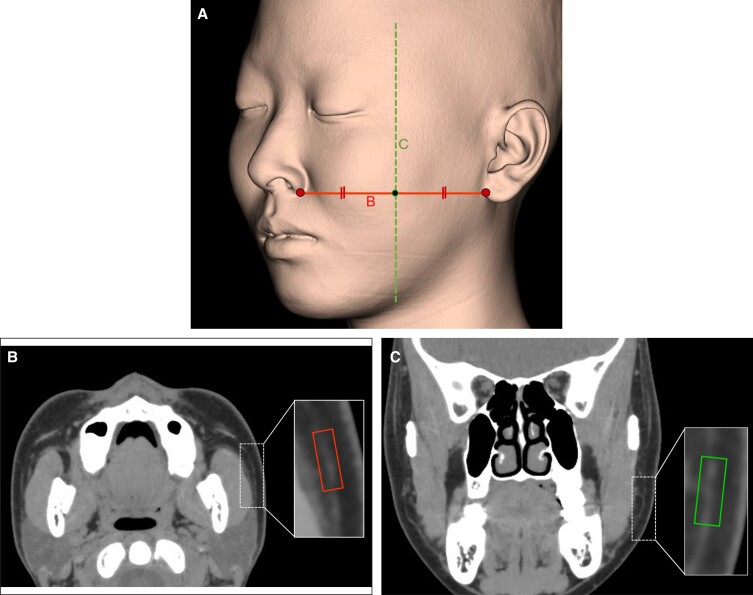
Quantitative analysis on axial and coronal computed tomography (CT) images of superficial musculoaponeurotic system (SMAS) analysis. (A) Three-dimensional (3D) CT image showing anatomical landmarks for standardization of the analysis site of the SMAS. The inferior margin of the nose and anterior margin of the earlobe were used as anatomical landmarks (large dots) for an axial CT image, and the center of the fixed analysis area (FAA; small dot) was installed at the midpoint connecting them. The horizontal line B indicates the location of the axial CT image. A coronal CT image was created at the location of the small dot in a plane orthogonal to the axial CT image along the vertical dotted line C. (B) Axial CT images for quantitative analysis of the SMAS: the subcutaneous adipose tissue containing the SMAS was delineated using a 9 × 3 mm rectangle, and this rectangular area was defined as the FAA. The subcutaneous adipose tissue of the cheek was sufficiently thick and determined to be optimal for SMAS analysis. Even within the FAA, SMAS thickness is not uniform. The area occupied by the SMAS within the FAA was quantitatively analyzed using ImageJ software with contrast enhancement and binarization. (C) Coronal CT images for quantitative analysis of the SMAS. Using the same method as for the axial CT image, the area occupied by the SMAS within the FAA was quantitatively analyzed using ImageJ software (National Institutes of Health).
